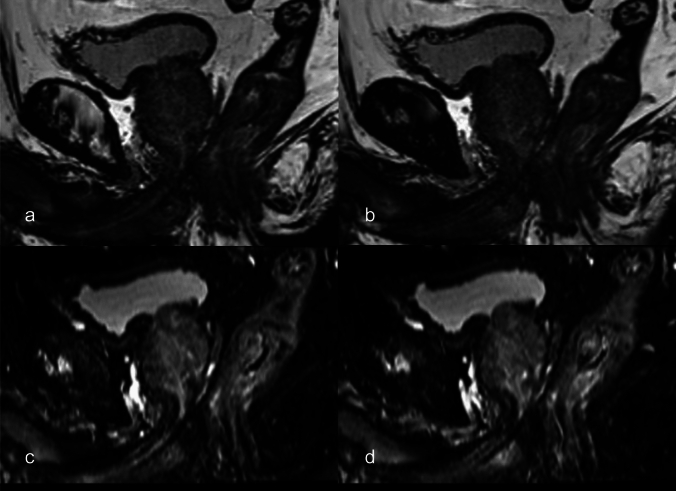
Images of a 75-year-old male in Case 10. The DSC value was 0.59 in the conventional 3D T2-weighted image (a, b) and 0.64 in the proposed fat-suppressed 3D T2-weighted image (c, d). The visibility score was 2 in both. This was the only case in which the prostatic urethra was obscured; this patient had benign prostatic hyperplasia, which may have been the cause
